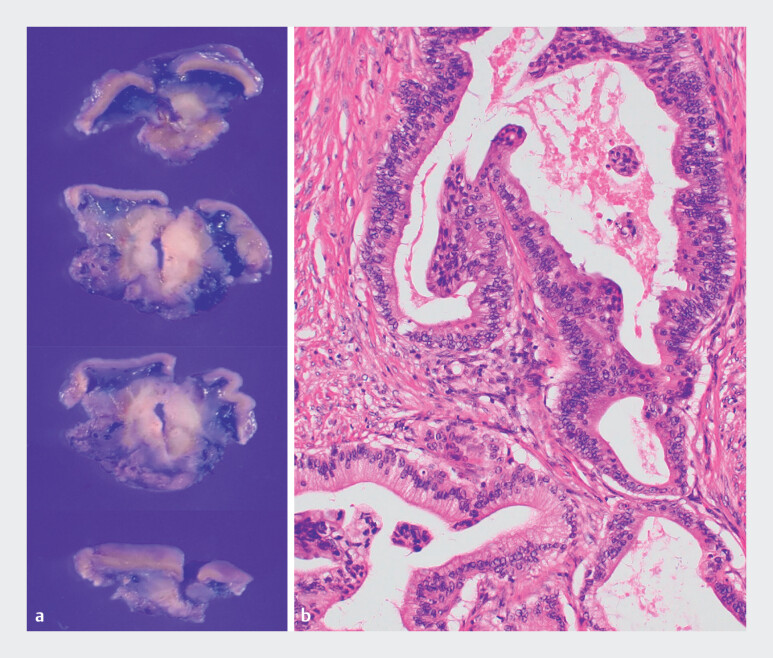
Surgical resection of needle tract seeding (NTS) after endoscopic ultrasonography-guided fine-needle aspiration (EUS-FNA): intraoperative clip-guided local resection and pathological confirmation.
